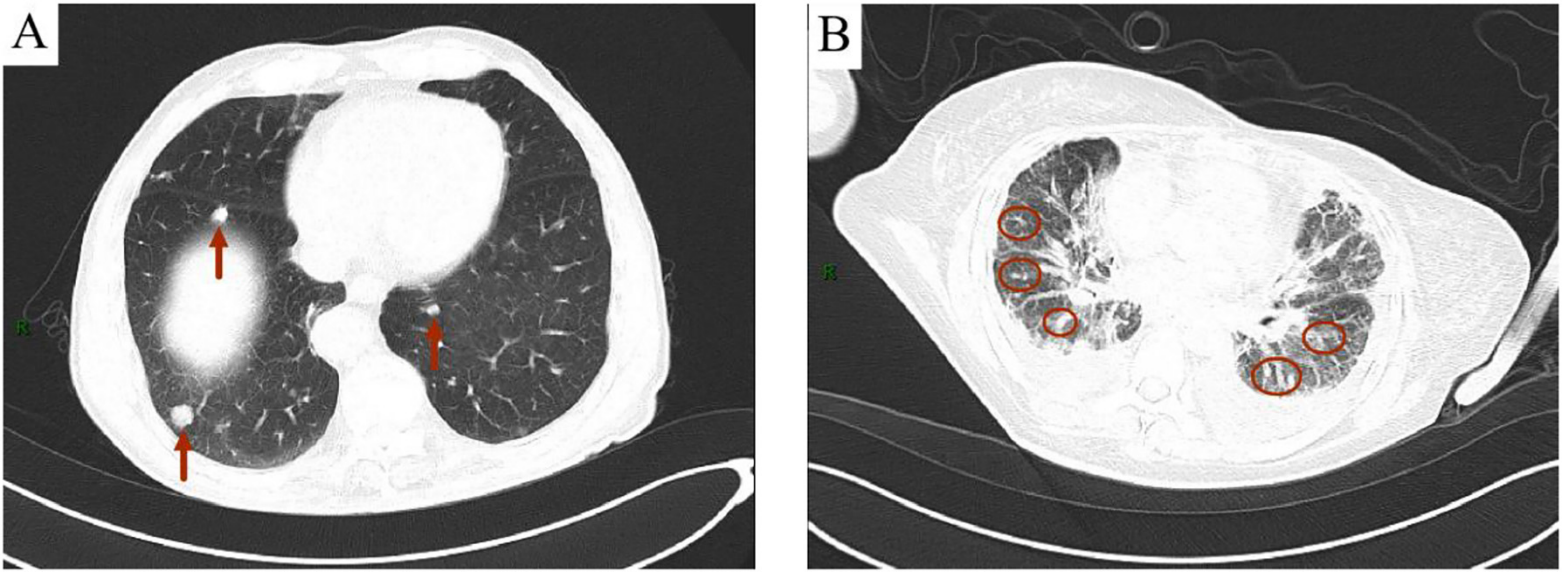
(Chest CT) (A) Case 1 multiple nodular and linear high-density shadows in both lungs (indicated by arrows); (B) Case 2 multiple patchy, linear, and nodular increased density shadows in both lungs, unclear boundaries (circled).
